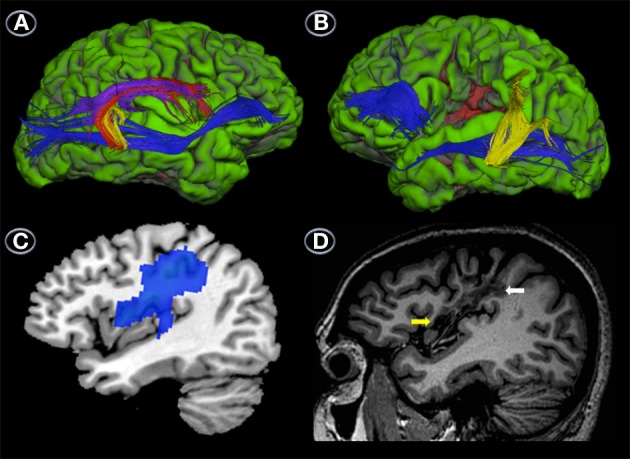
Uninflated surface of the right (A) and left hemispheres (B) (FreeSurfer reconstruction) of the patient with transcortical motor aphasia (RTP) showing gyri cultured in green with sulci shown in red. DTI of right hemisphere perisylvian pathways superimposed on RTP anatomical MRI shows well-developed temporo-frontal, temporo-parietal and parieto-frontal streams (red, yellow, and purple, respectively) and ventral stream (blue) (A), whereas in the left hemisphere only the parieto-temporal segment of the arcuate fasciculus (yellow) and part of the ventral stream (blue) could be reconstructed (B). This image also shows the location of the infarct in the lower pericentral region (red area). Parasaggital T_1_-weighted MRI view of the left hemisphere shows the infarction in the sensorimotor cortex (white arrows) and medial insula (yellow arrow) (C). Parasaggital view of the ^18^FDG-PET (MRIcroN) showing an area of reduced metabolic activity in the left fronto-insular region (blue) (D) which is slightly larger than the area of infarction depicted in (C). See further details in Table 4.
